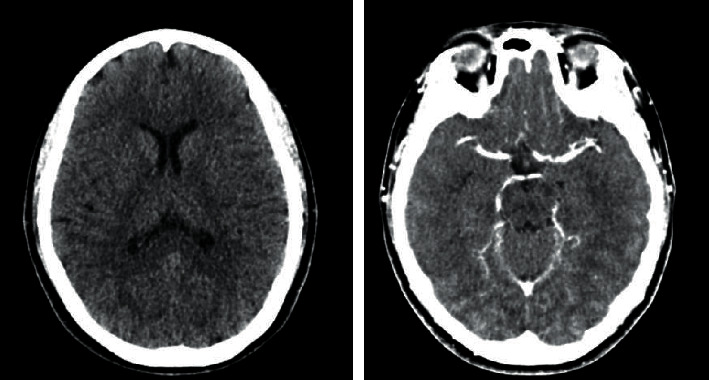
Brain computed tomography and venography revealed diffuse absence of cortical sulci without venous obstruction, consistent with diffuse cerebral edema.
